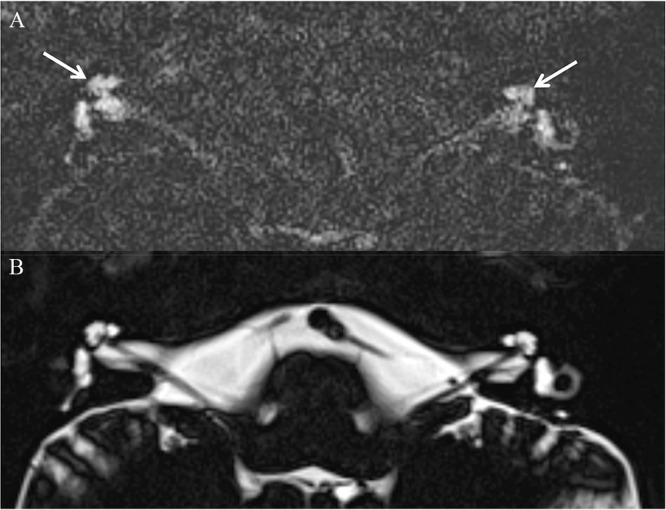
A 31-year-old male volunteer. (A) Heavily T_2_-weighted three-dimensional fluid-attenuated inversion recovery (3D-FLAIR) image obtained 4 hours after intravenous administration of a single dose of gadolinium-based contrast agent (IV-SD-GBCA). High signal is seen bilaterally in the cochleae (arrows). In this particular subject, the signal intensity ratio (SIR) was 7.8 in the right cochlea and 7.5 in the left cochlea. (B) magnetic resonance cisternography (MRC) at the slice level corresponding to that in (A).
